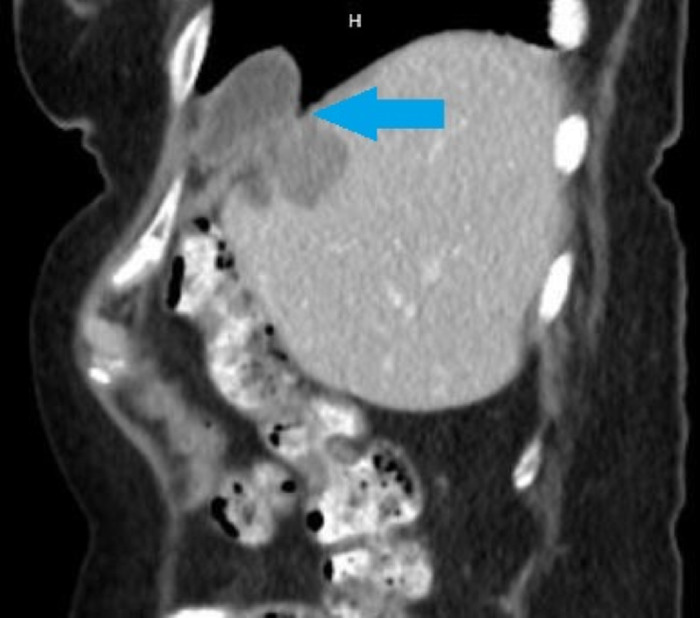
Computed tomography sagittal view shows gallbladder diaphragmatic herniation (arrow).
